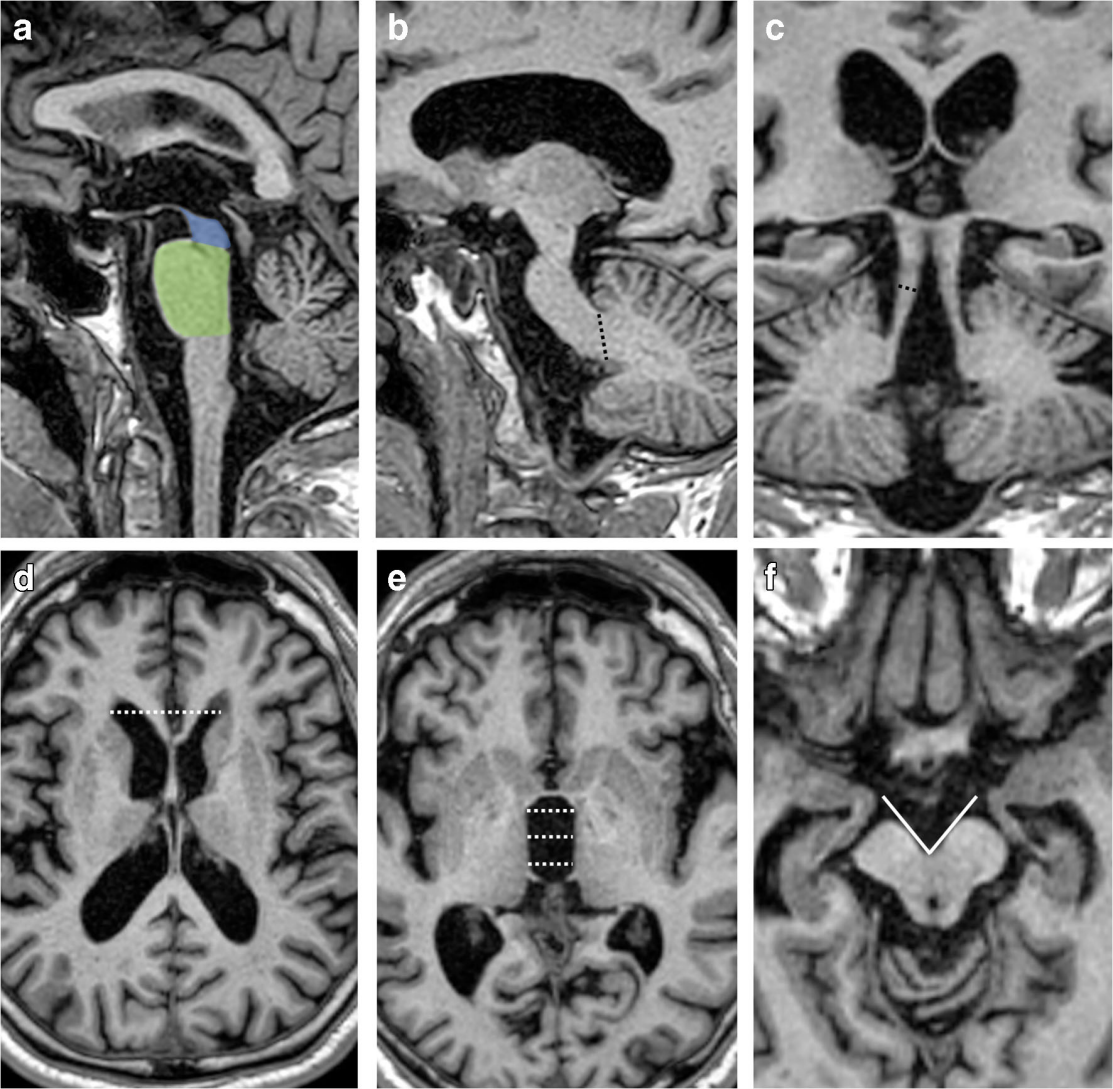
Sagittal (a–c) and axial (d–f) T1-weighted volumetric MR images of a PSP patient
